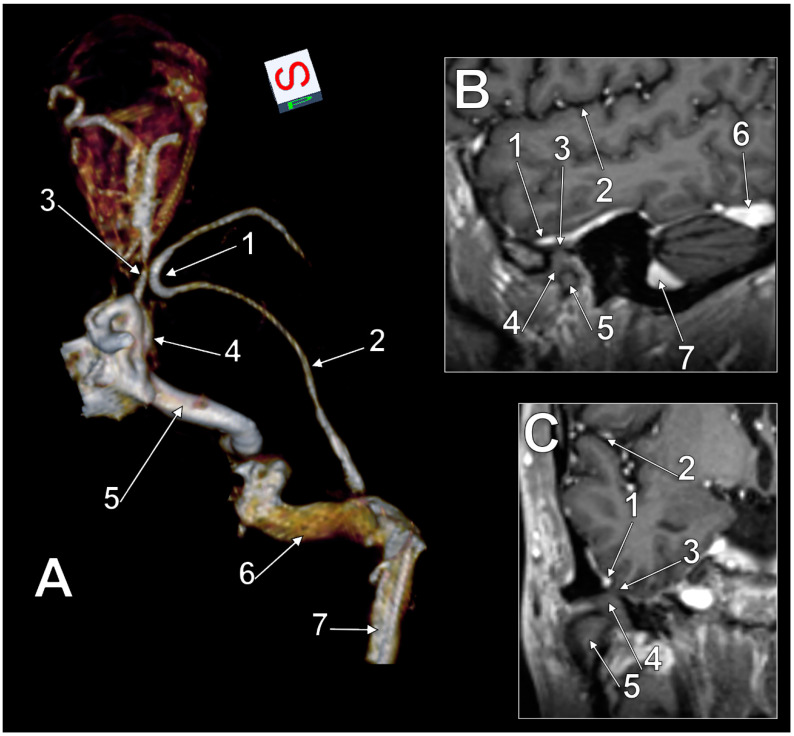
Three-dimensional volume rendering of (A) the right superficial middle cerebral vein (SMCV). Postero-supero-medial view. 1. coronal loop of the SMCV; 2. basal course of the SMCV; 3. superior ophthalmic vein; 4. cavernous sinus; 5. internal carotid artery; 6. sigmoid sinus; 7. transverse sinus. (B) Sagittal and (C) coronal MRI slices through the right temporomandibular joint. 1. superficial middle cerebral vein of the basal type; 2. Sylvian fissure; 3. dehiscent mandibular fossa of the temporal squama; 4. articular disc; 5. mandibular condyle; 6. transverse sinus; 7. sigmoid sinus.
